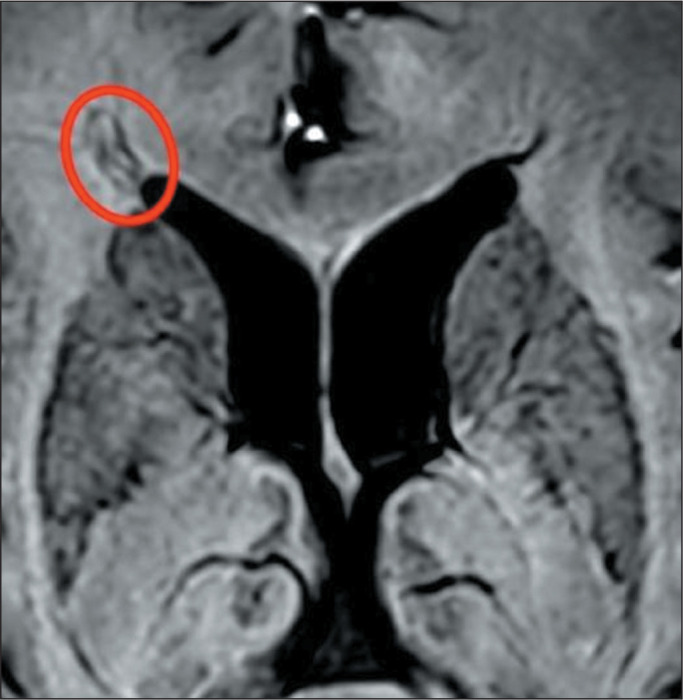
Axial T2-weighted SWI magnitude image: hypointense iron deposit ring
